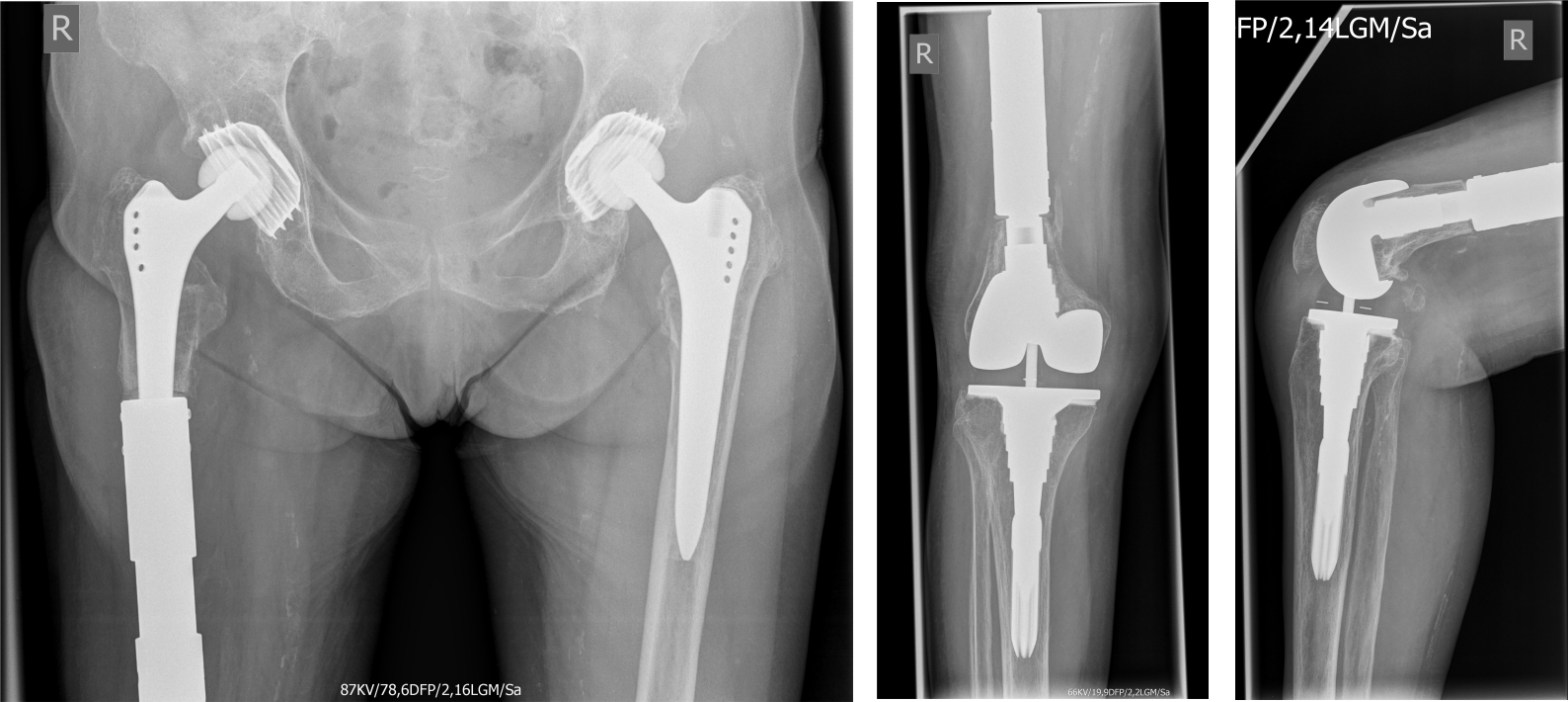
Postoperative radiographs one year after surgery without any morphological changes compared to postoperative radiographs
